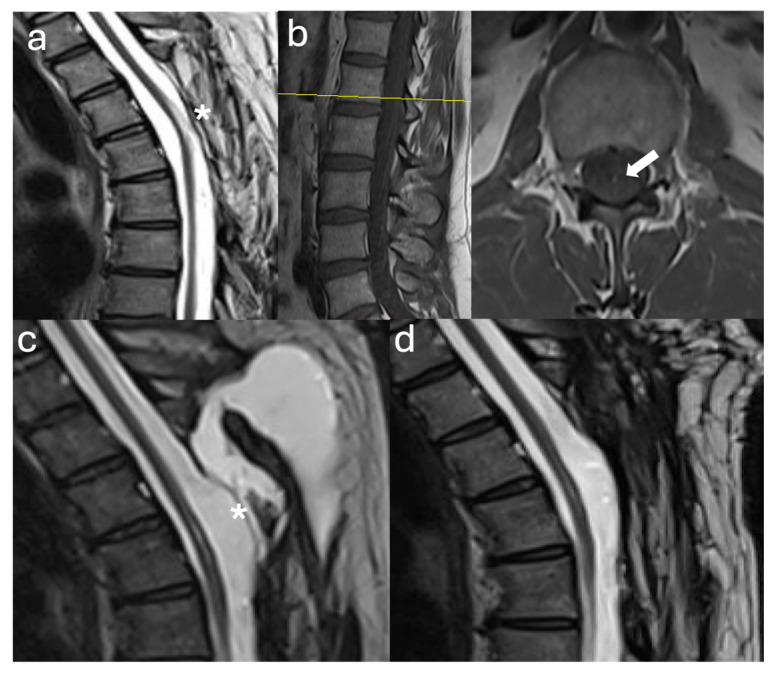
Radiographic Images Case 3: (a) Preoperative sagittal thoracic MRI T2 sequence shows fusiform cord atrophy with expansion of the epidural space and tethering of the spinal cord to the dura at the prior tumor resection cavity at the T4–5 level (asterisk). (b) Preoperative lumbar MRI T1 sequence shows a prominent and hyperintense appearing filum terminale on sagittal and axial sequences below the conus level that terminates at L1 (yellow line, arrow on axial sequence). This is suspicious for a dysplastic conus/filum transition. (c) Postoperative sagittal thoracic MRI T2 sequence shows a patulous cerebrospinal fluid space with complete detethering of the spinal cord after expansile duraplasty (asterisk) and a T3–T6 sizeable midline fluid collection posterior to the duraplasty consistent with a pseudomeningocele. (d) Thoracic MRI T2 sequence after the repair of the pseudomeningocele shows complete resolution of the dorsal fluid collection.
